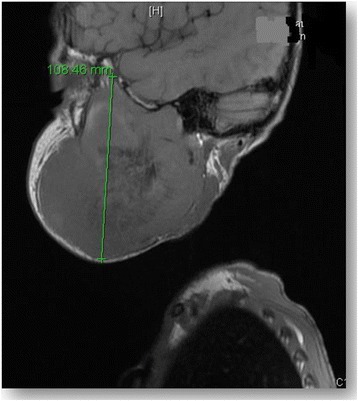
MRI of the mandibular area revealed a large necrotic and heterogeneous right mandibular soft tissue mass with extension into the infratemporal fossa (arrow)
